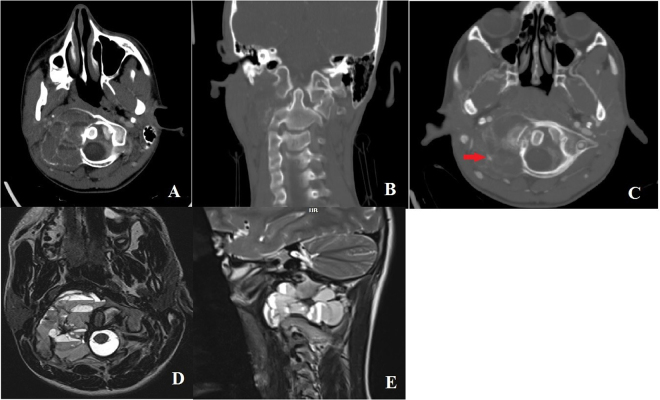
(A) an axial CT scan showing an expansile lesion with septations in an egg-shell appearance involving the atlas lateral mass, with anterior and posterior arc destruction and retropharyngeal extension. (B) a coronal CT revealed destruction of the right side lateral mass and C1-C2 dislocation on the left side. (C) the right VA is encased by the lesion based on CT-angiography of VAs (red arrow). (D) axial T2-weighted MRI demonstrates a cystic lesion with a fluid-fluid appearance without evidence of cord compression. (E) the sagittal view depicts the anterior-posterior extension of the tumor to the retropharyngeal space.
